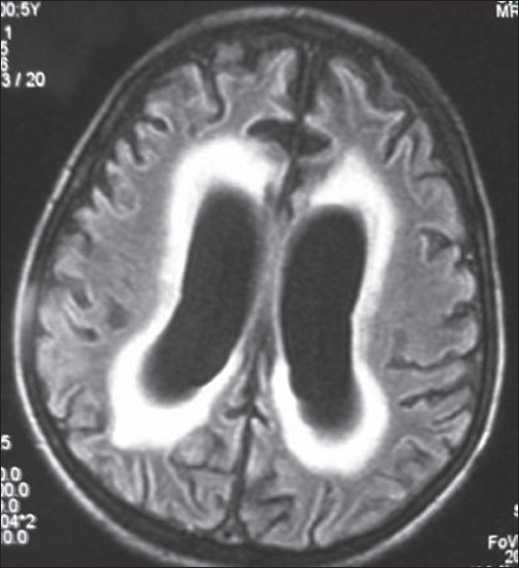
Cranial MRI shows ventricular dilatation and cortical atrophy
